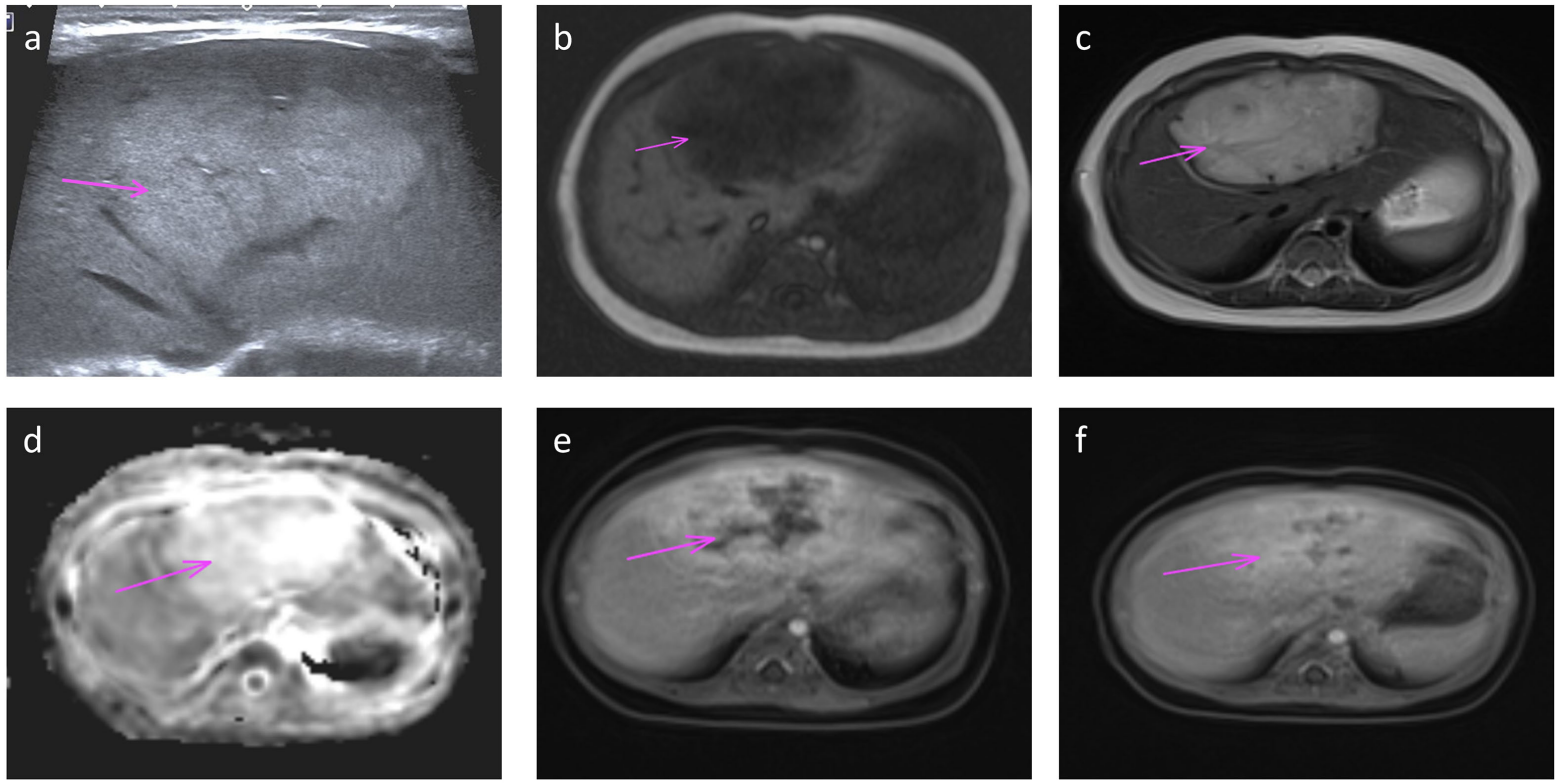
Ultrasound (a) and MRI (b-f) performed at one month of age shows a large ovoid mass in the left lobe (arrow). Transverse ultrasound image (a) of the left lobe shows homogeneous increased echotexture. MR axial T1 and T2 weighted images (b, c) show low T1 and high T2 signal with vascular flow voids on T2; high signal on diffusion ADC map (d); and centripetal filling on dynamic-enhanced images with peripheral enhancement in the arterial phase (e) and progressive filling in the delayed phase (f).
